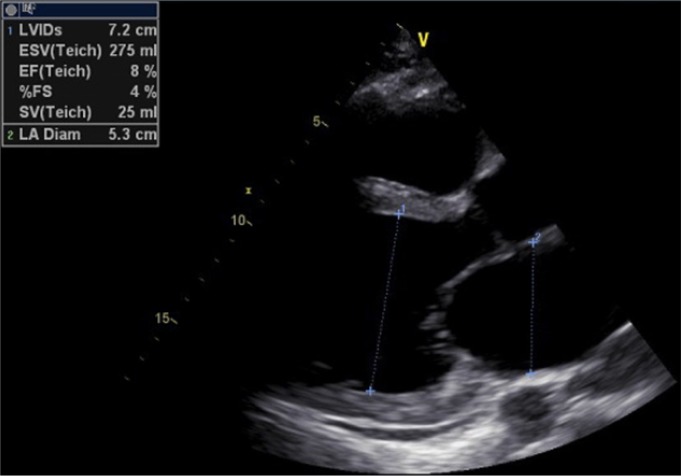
Transthoracic echocardiography of the patient (parasternal long axis view)
